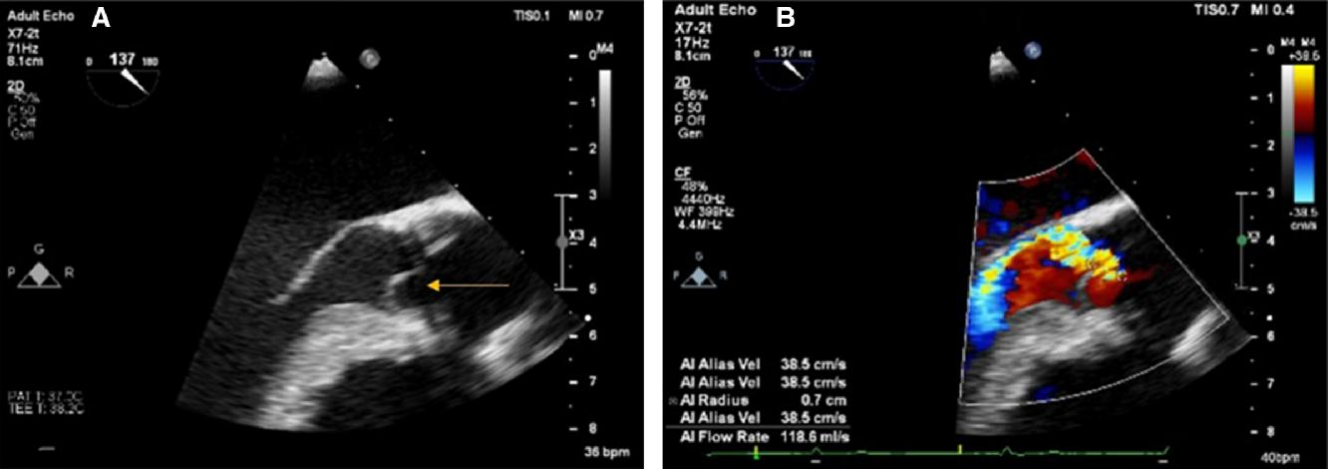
Transoesophageal echocardiography (long axis view) demonstrating recurrence of aortic regurgitation (AR) after valve sparing aortic root replacement due to prolapse of the right coronary cusp (arrow—*A*) and with colour Doppler (*B*) showing an eccentric jet of severe AR (vena contracta 0.7 cm).
